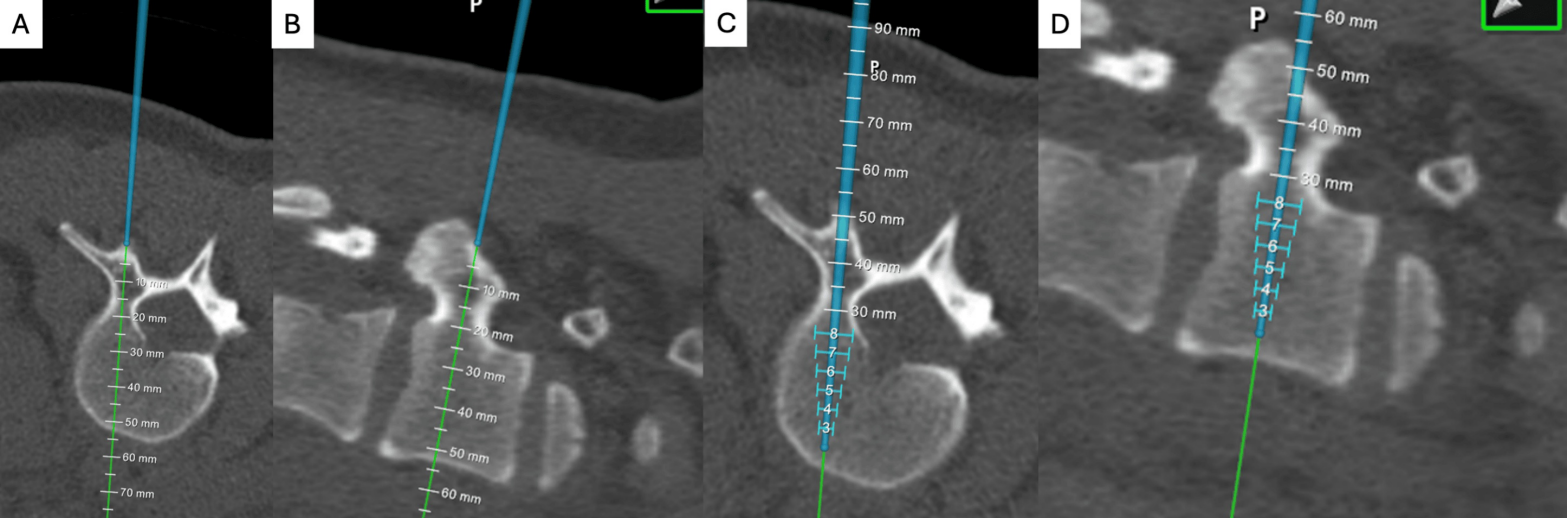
(A) A navigated probe is used to map the optimal trajectory of a pedicle screw on an axial slice of the preoperative CT scan after identifying anatomic landmarks with a traditional freehand technique. (B) A navigated probe is used to map the optimal trajectory on a sagittal slice of the preoperative CT scan. (C) A navigated awl with projected screw lengths overlaid onto an axial slice of the preoperative CT scan. (D) A navigated awl with projected screw lengths overlaid onto a sagittal slice of the preoperative CT scan. CT: Computed tomography
